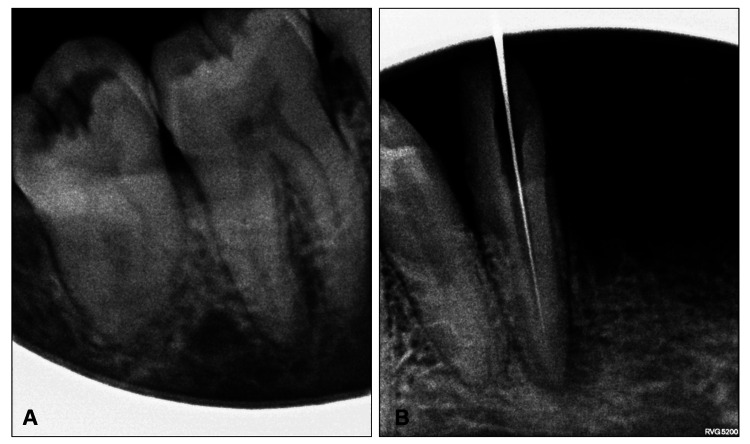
(A and B) Intraoral periapical radiographic images taken using a wall-mounted device
